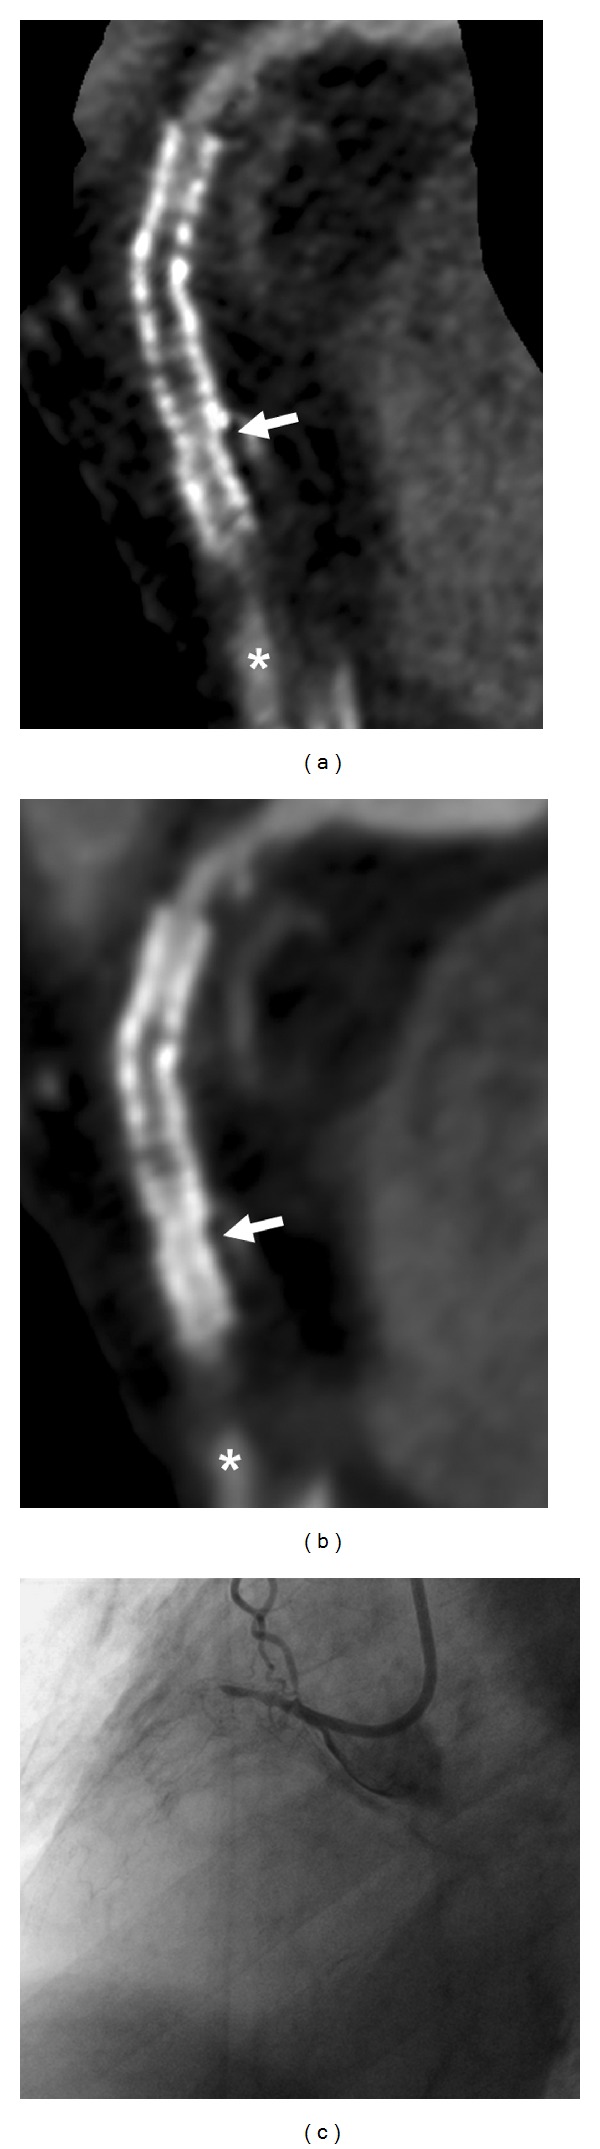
73-year-old male patient with a history of myocardial infarction and percutaneous recanalization of the RCA with implantation of a 3 mm Coroflex blue and 3 mm Vision stent. CT images computed with a dedicated convolution kernel (a) and a smooth kernel (b) show total stent occlusion with distal filling of the vessel (asterisk) via collateral flow. This finding was confirmed by conventional coronary angiography (c). CT also showed a step with incongruent course of the stents, indicating stent fracture.
